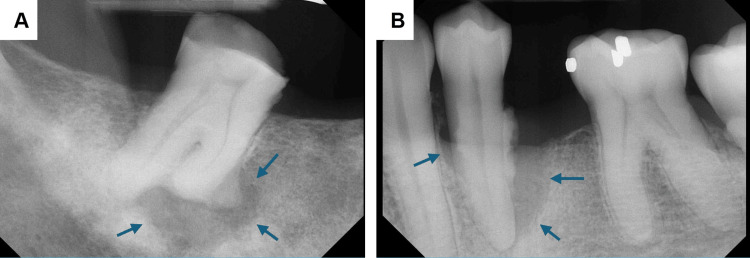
Radiograph showing (A) periapical lucency of tooth #31 and (B) radicular lucency of tooth #17 Blue arrows are pointing to the lucency associated with the teeth.
